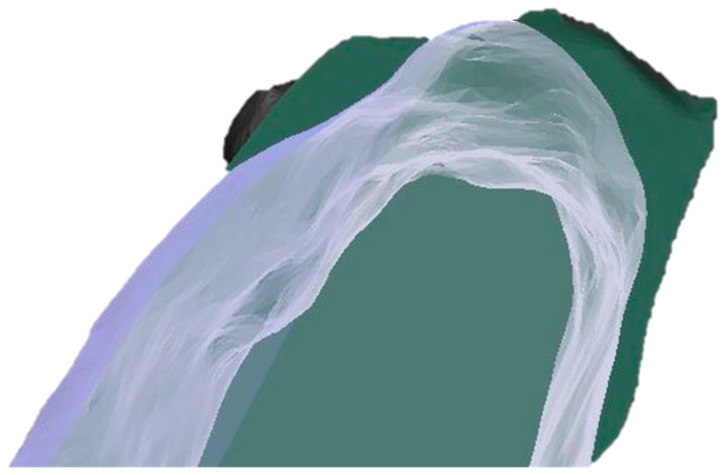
A visual example of the generated CBCT superimposition showing a comparison of the baseline and 4 months post alveolar ridge preservation at the test site (dark green, initial state; transparent white, 4 months post-op).
